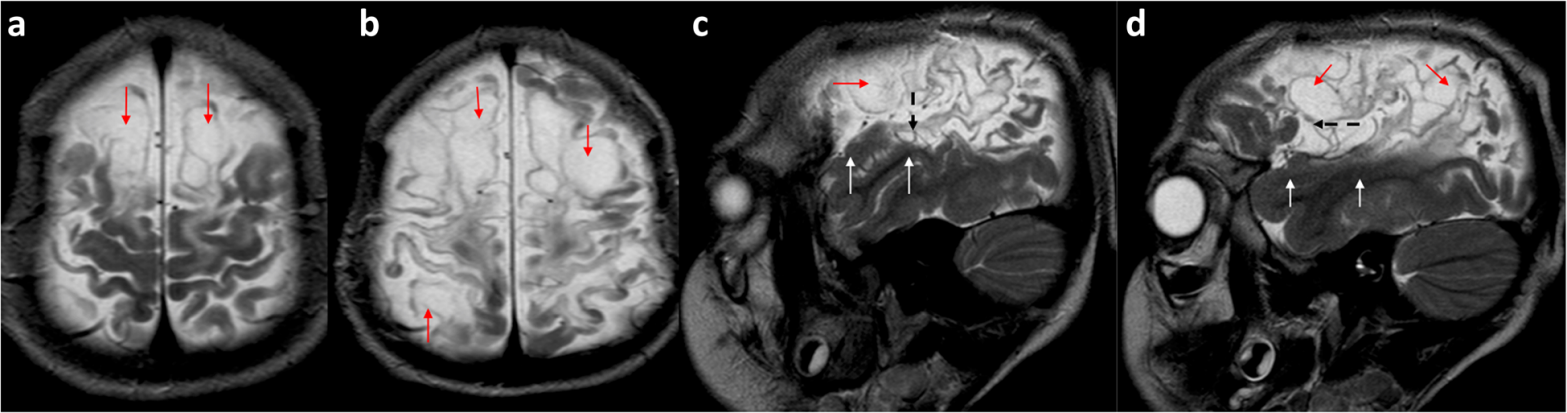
a–d T2-weighted imaging of a 6-year-old boy who sustained a severe/catastrophic prolonged hypoxic-ischaemic injury at term gestation. Axial image just below the vertex (a) and sagittal image of the right lateral peri-sylvian region (b) shows extensive injury of the watershed continuum represented by multi-cystic encephalomalacia (red arrows). Of note, c and d are the extension of peri-Sylvian involvement into the posterior temporal lobe and extension of the parasagittal cystic encephalomalacia up to the Sylvian fissure (dashed black arrows) but with preservation of the superior temporal gyrus anteriorly seen on the sagittal images (solid white arrows)
